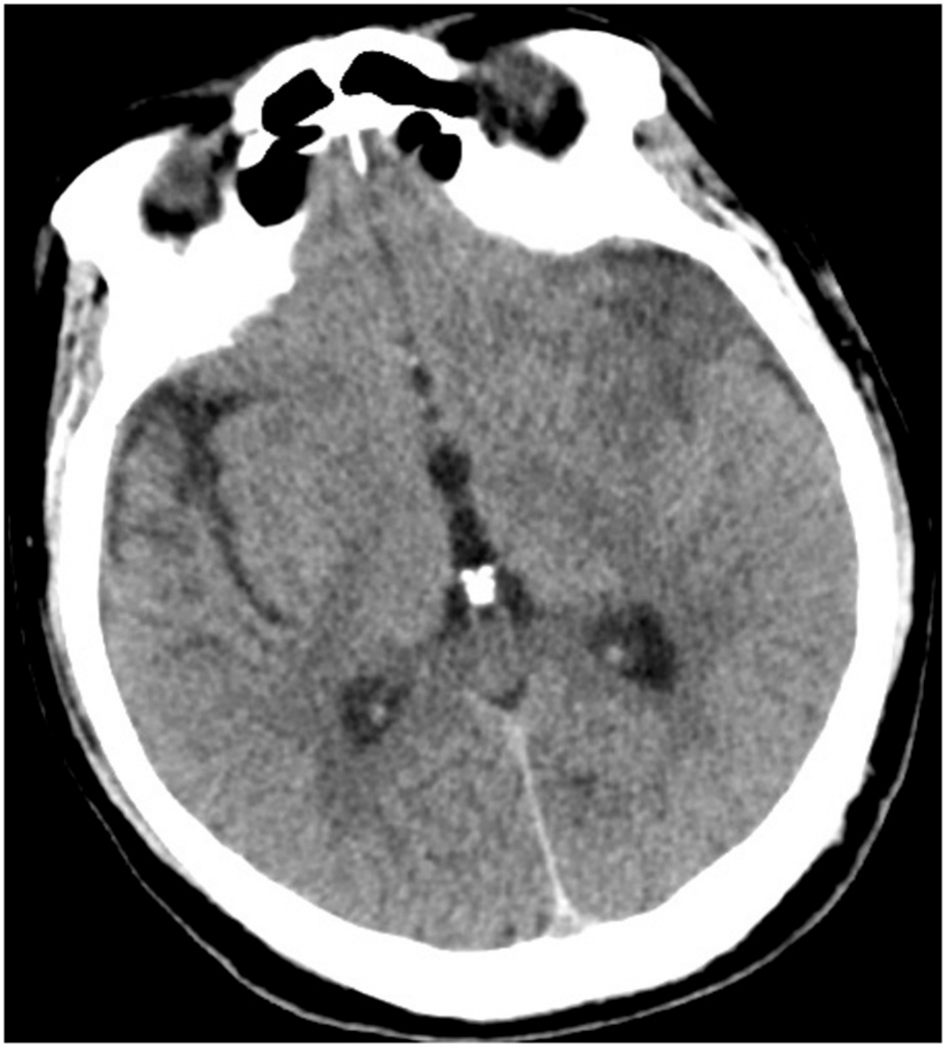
Initial head CT revealed left frontal lobe and left insular lobe hypodensities.
